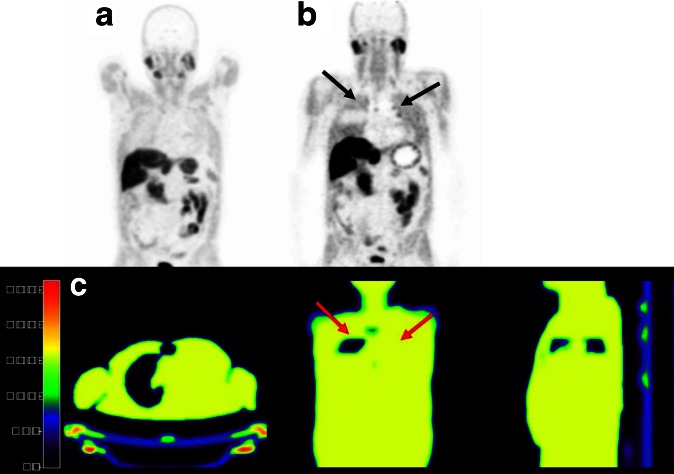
Impact of incorrect lung segmentation: a PET image using CT-based attenuation correction; b PET image using MRI-based attenuation correction, showing artificially increased uptake in the most of the lung tissue (black arrows); and c corresponding MRI-based attenuation map with incorrect lung segmentation (*red left arrow*: segmentation of only the apex of the right lung; *red right arrow*: no segmentation of the left lung).
